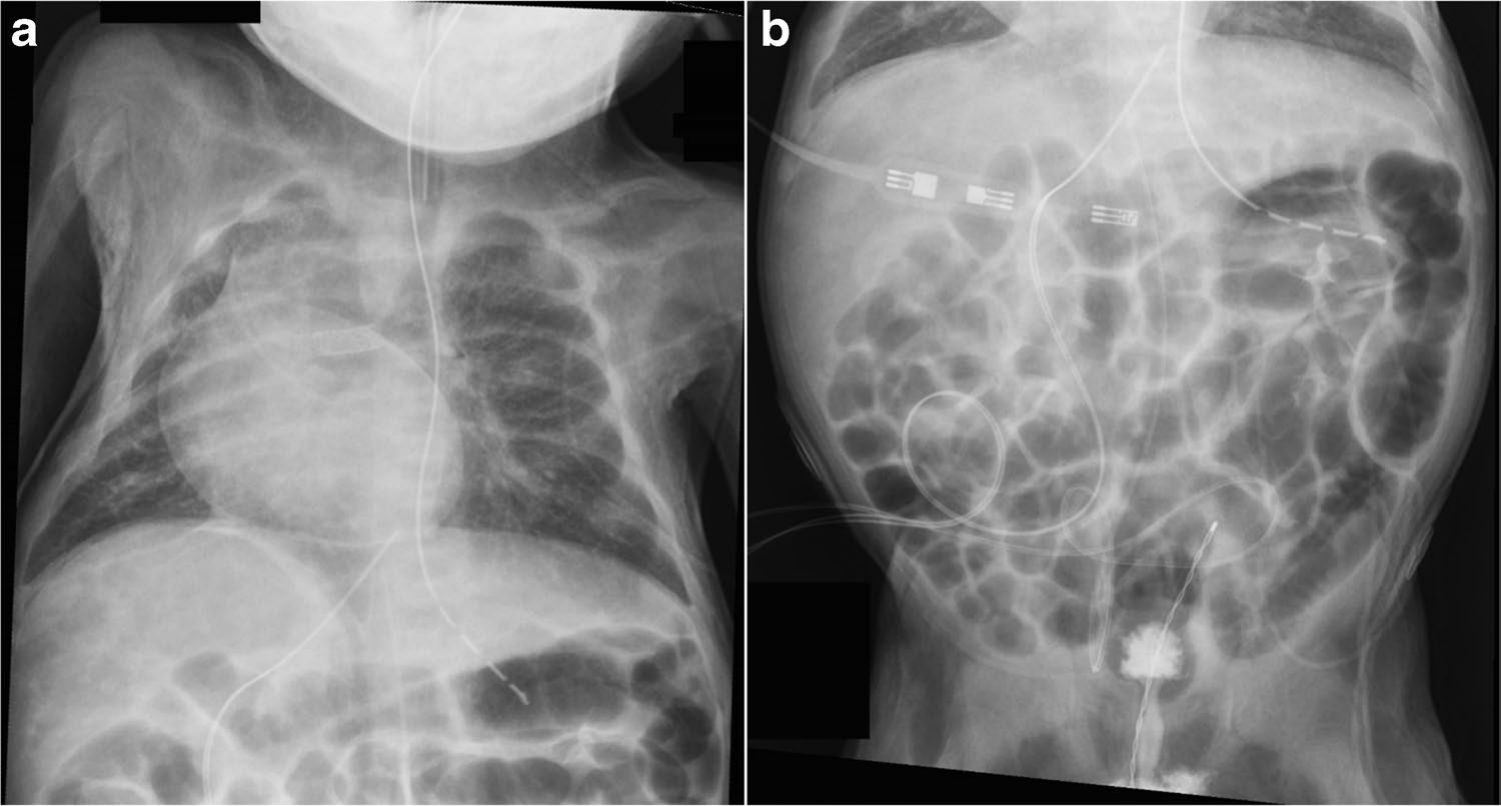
a Chest radiograph of a 6-day-old boy obtained 217 min after stent placement for a patent ductus arteriosus shows increased density in the subcutaneous soft tissues and the pericardium. The increased density causes the liver, heart, and some subcutaneous fat to appear more lucent than normal. This patient was misdiagnosed with pneumoperitoneum. b Subsequent abdominal radiograph obtained 131 min later shows increased density of the subcutaneous tissues and relative lucency of the liver. A cross-table lateral radiograph (*not shown*) confirmed that pneumoperitoneum was not present
